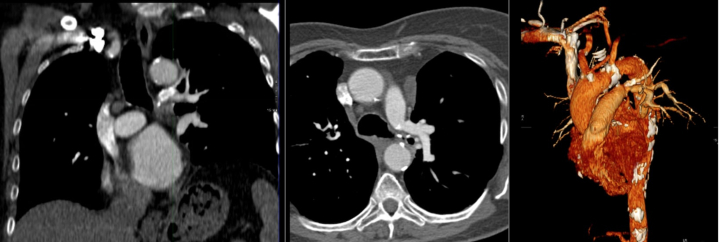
Follow-Up Thoracic Computed Tomography Angiography Showing Stent Patency 7 Years After the Acute Episode
